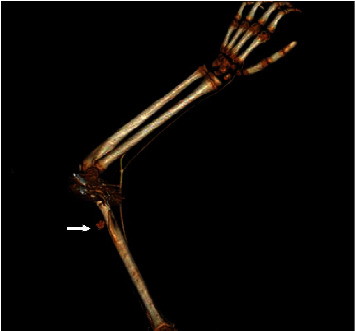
3D reconstruction of CT angiography demonstrating a pseudoaneurysm of profunda brachii artery with extravasation of contrast (white arrow).
